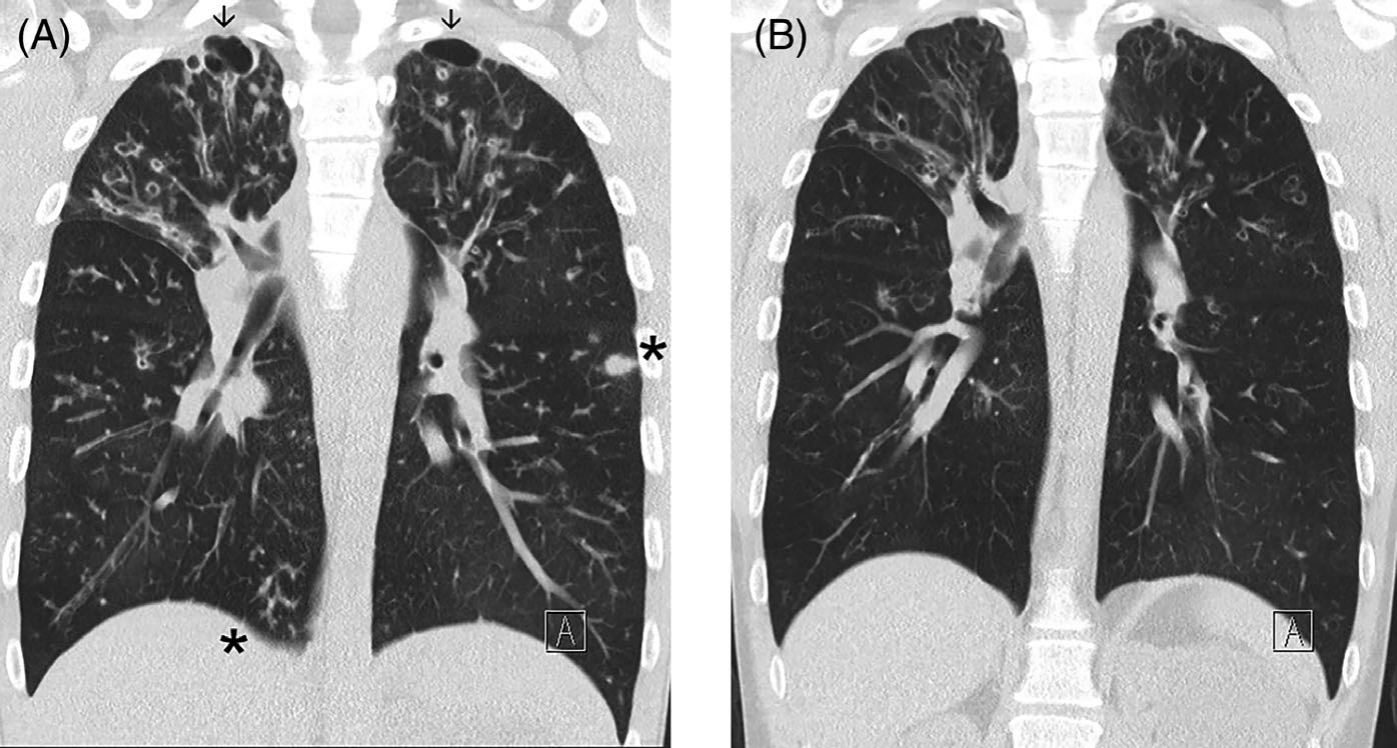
CT scans (A) before and (B) after elexacaftor + tezacaftor + ivacaftor for case 2
